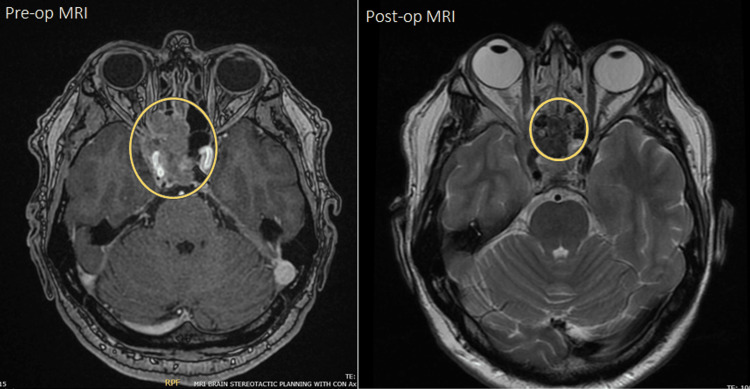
Preoperative and postoperative MRI of the brain.
